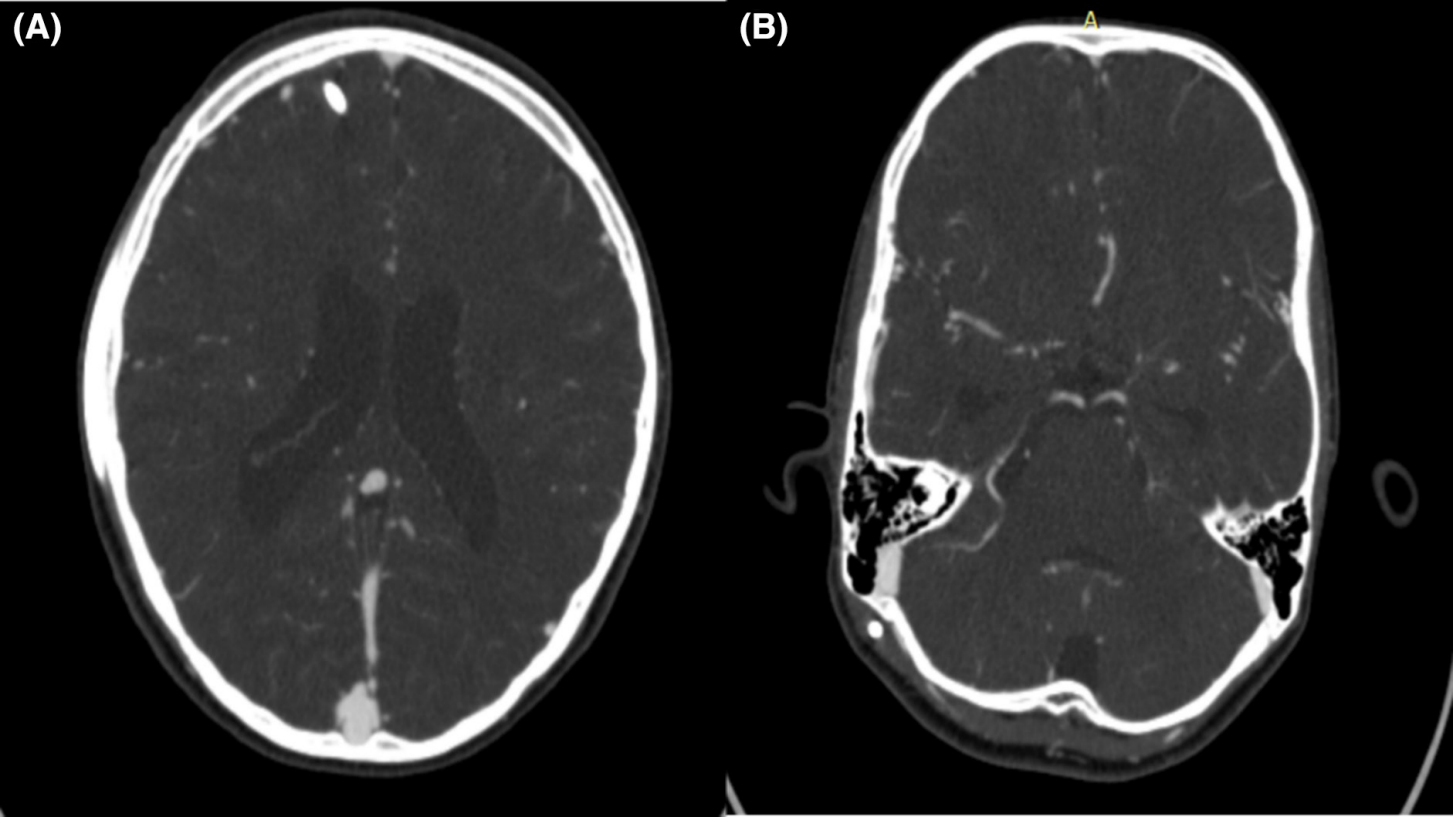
(A, B) CT angiogram taken after resolution of bleed 2 months after discharge.
